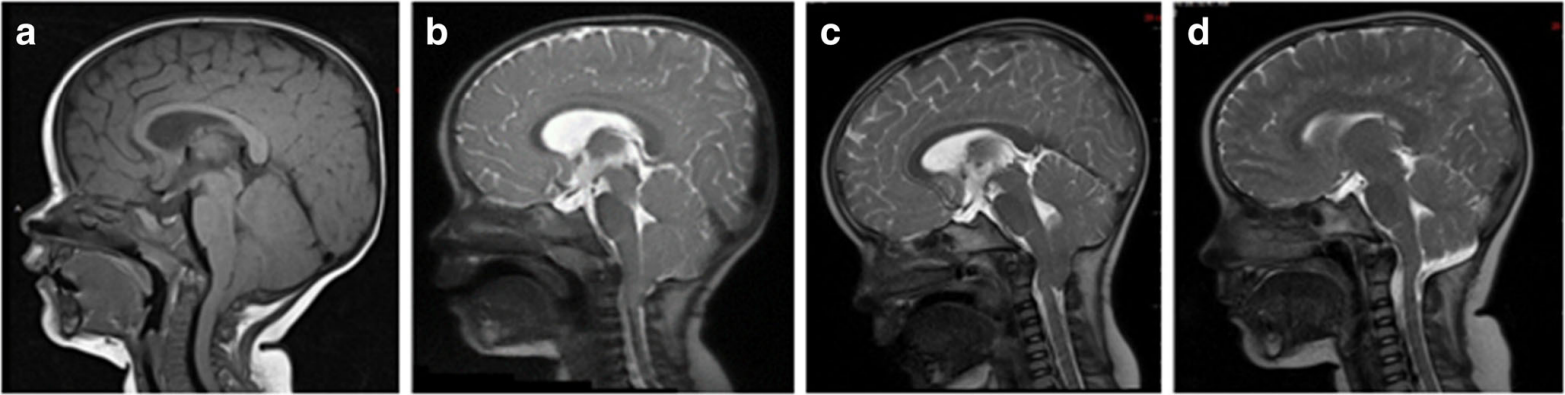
An 11-month-old girl (Case 3) presented initially with increasing snoring and coughing during the sleep, and more recently, the patient showed irritability with holding the back of her head. Midsagittal T1-weighted MRI (A) showed Chiari I malformation with tonsils herniating up to above portion of the C2 lamina. There is an abnormal intensity in the upper cervical cord. She underwent posterior fossa decompression and dural scoring(Type 1 procedure) and postoperatively all her symptoms resolved. Midsagittal fast T2-weighted MR (B), 3 months after the decompression, showing little improvement in the decompressed foramen magnum. Three years later, she developed grabbing occiput, nighttime waking, and snoring. Midsagittal fast T2-weighted MR (C) showing a tight posterior fossa and upper cervical space with medullary compression. A reoperation with tonsillar thermal reduction and dural patch graft was performed. Her snoring promptly resolved and the latest MR done 2 years after the second operation showed a good decompression (D)
